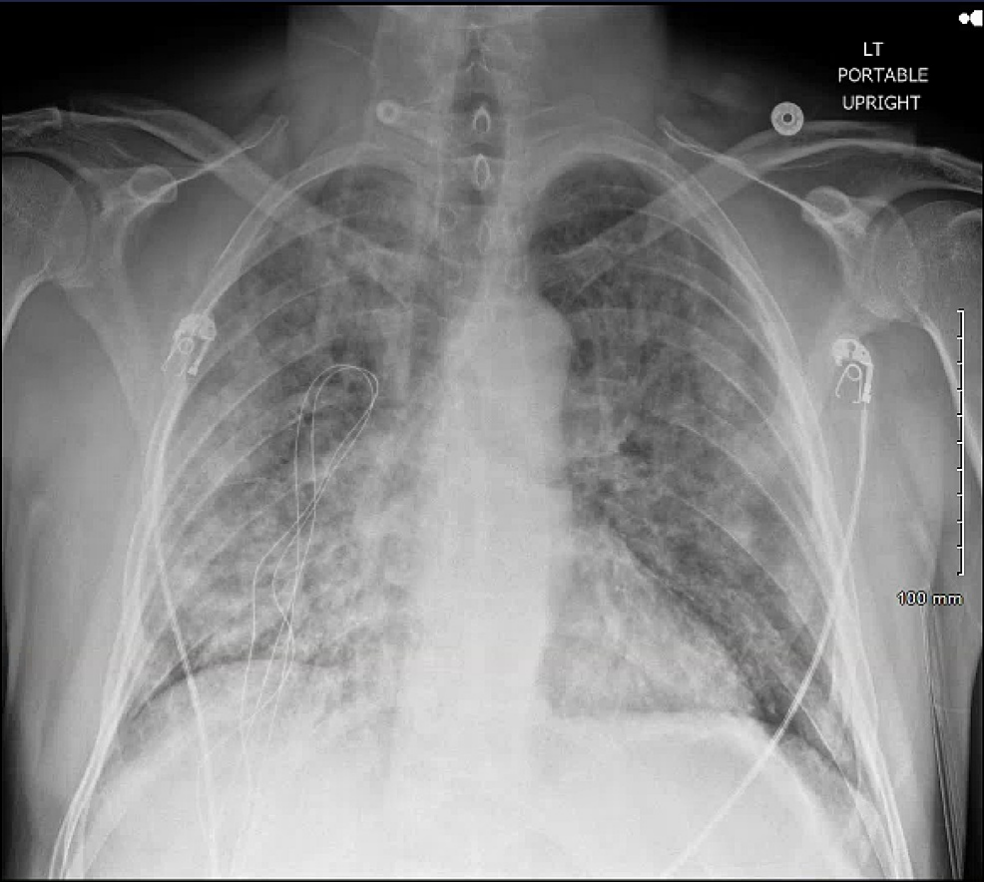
Chest radiograph demonstrating diffuse bilateral opacities.
